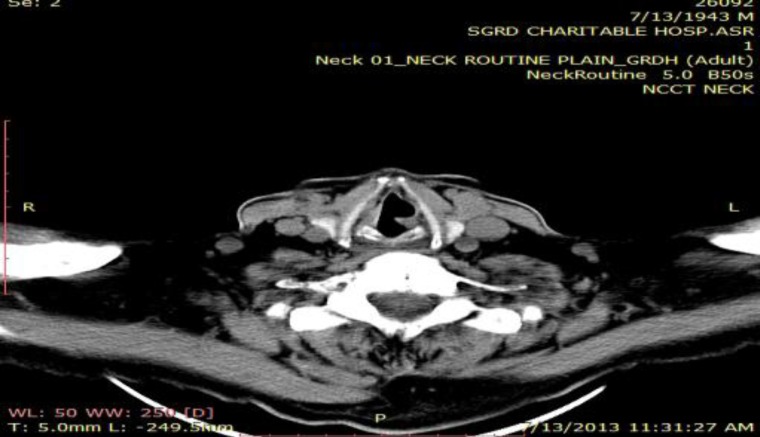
Contrast Enhanced computed tomography scan shows atrophy of the left thyroarytenoid muscle with enlargement of the left laryngeal ventricle indicating left laryngeal nerve palsy
